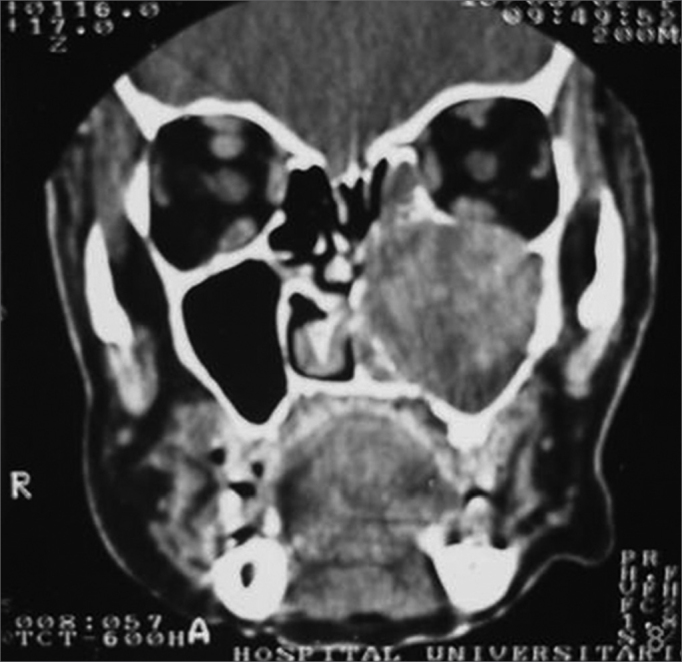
CT coronal slice, soft tissue window.
